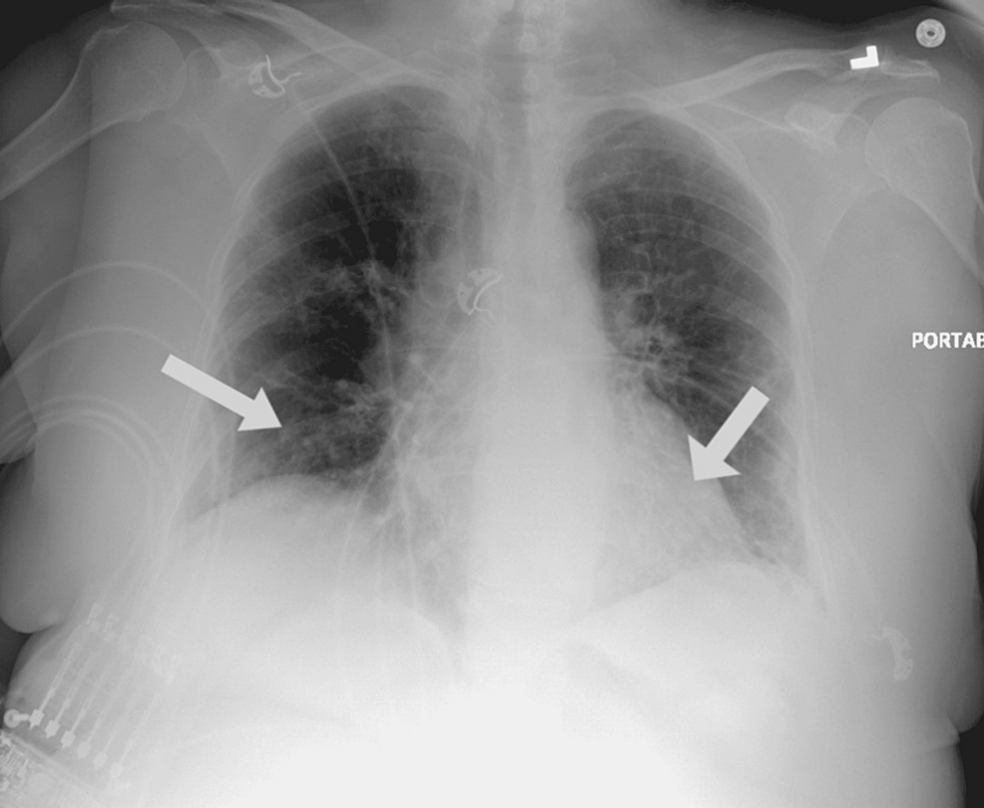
Chest x-ray: bilateral patchy opacities (white arrows) consistent with COVID-19 pneumonia. COVID-19: coronavirus disease 2019
